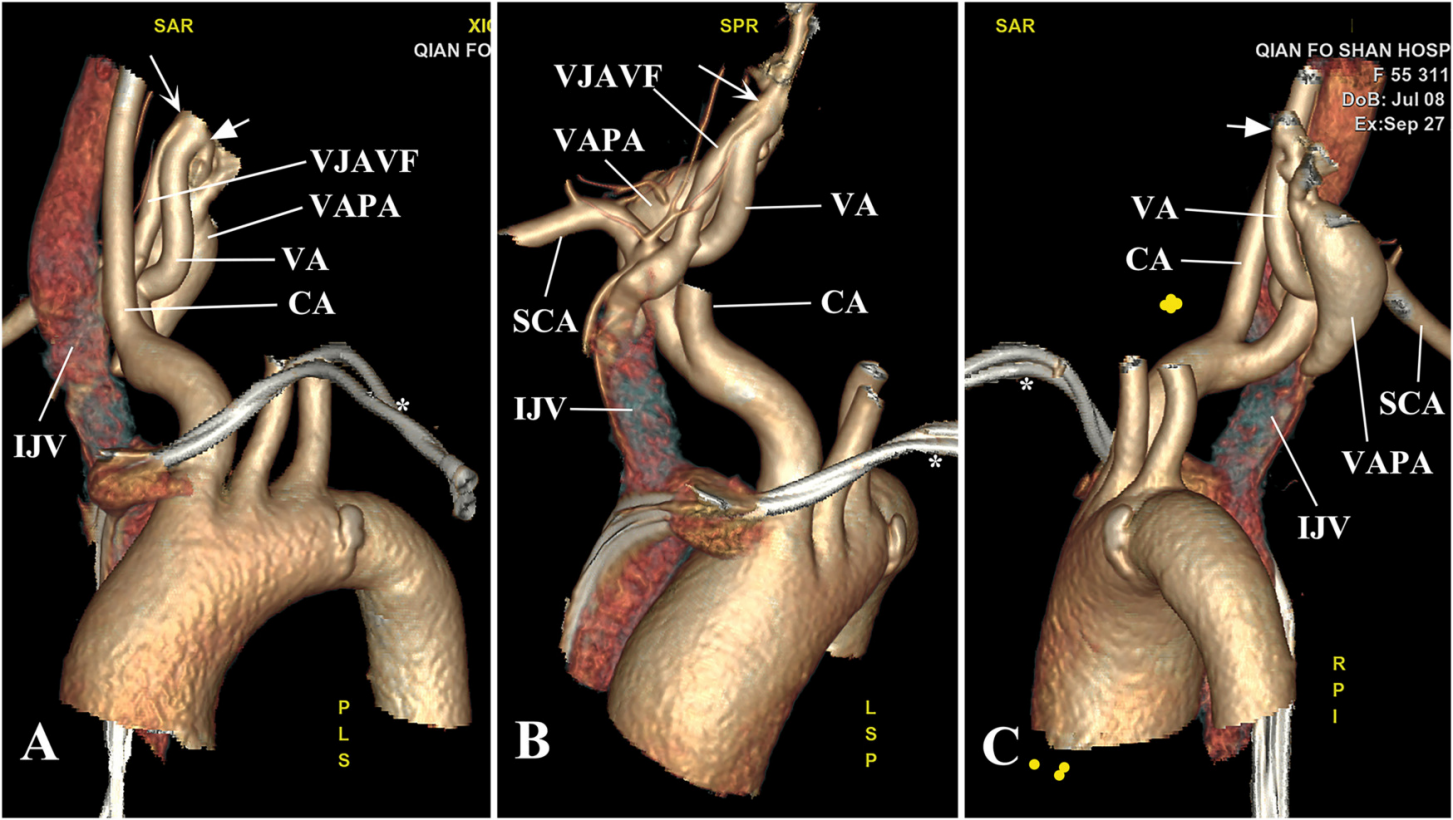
Anatomic location of the VJAVF and VAPA. (A) CTA revealed a VJAVF originating from the V1 segment of the right VA (arrow) that went down deeply behind the right CA and drained into the right IJV from behind. (B) The long course of the VJAVF is shown. (C) A banana-like VAPA, measuring 38 mm × 17 mm in size, originated from the opposite wall of the right VA (arrowhead) and extended down to the level below the right SCA without a drainage vessel. *indicates pacemaker wires. CA, carotid artery; IJV, internal jugular vein; SCA, subclavian artery; VA, vertebral artery; VAPA, vertebral artery pseudoaneurysm; VJAVF, vertebrojugular arteriovenous fistula.
